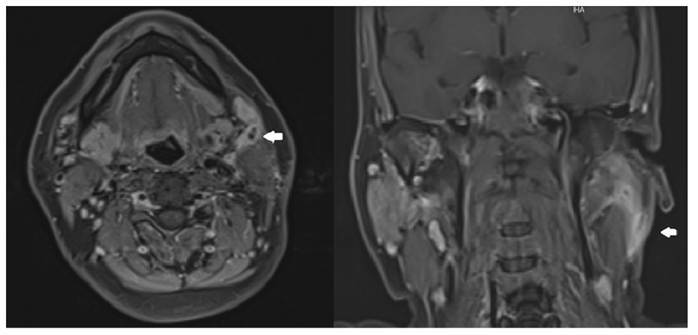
Contrast-enhanced neck computed tomography showing multiple necrotic lymph nodes and thickened, hyper-enhanced skin (white arrows).
